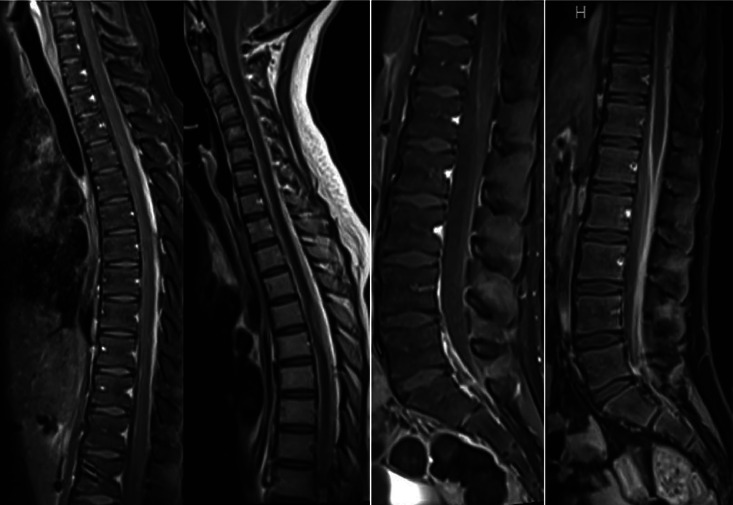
MRI, using T1-weighted and T2-weighted sequences in the sagittal plane of the cervical, thoracic and lumber spine shows the progression of the disease within 3 months (September-November). Significant progression of previously shown leptomeningeal metastases posteriorly (image on the left) and more to the left side extending from T4 down to T10 vertebral body (image on the right). There is an invasion of the posterior lateral aspect of the spinal cord on the left side with more than 50% of the cord involvement at the levels of T5 and T6, with the largest localized lesion measuring 1 × 0.9 cm in axial diameter and extending for 4.2 cm in cranial-caudal dimension compared to 0.6 × 0.7 × 3.6 cm on prior examination. There are also increased leptomeningeal metastases in the cervical, lower thoracic, and lumbar nerve roots, as well as the thecal sac, compared to prior examination. No aggressive bony lesions were seen. There is an increased degree of invasion of the spinal cord mainly on the left side extending from T4 down to T10.
